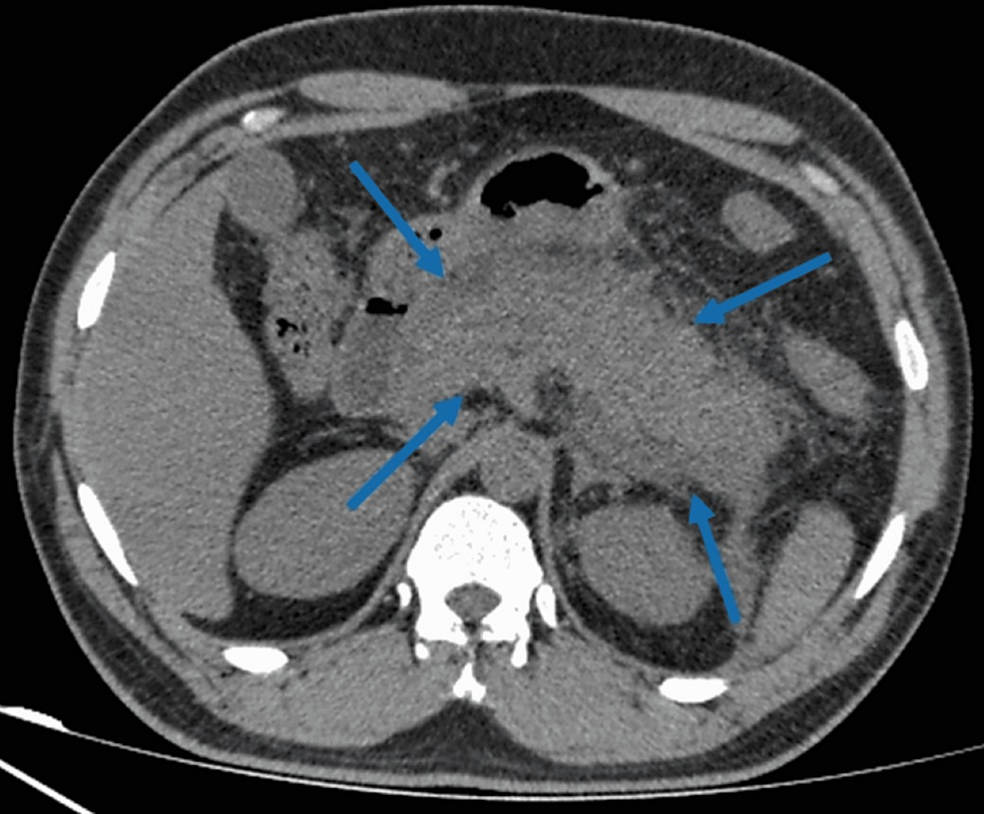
CT abdomen demonstrating diffuse inflammation of the pancreas with ill-defined borders (blue arrows).
